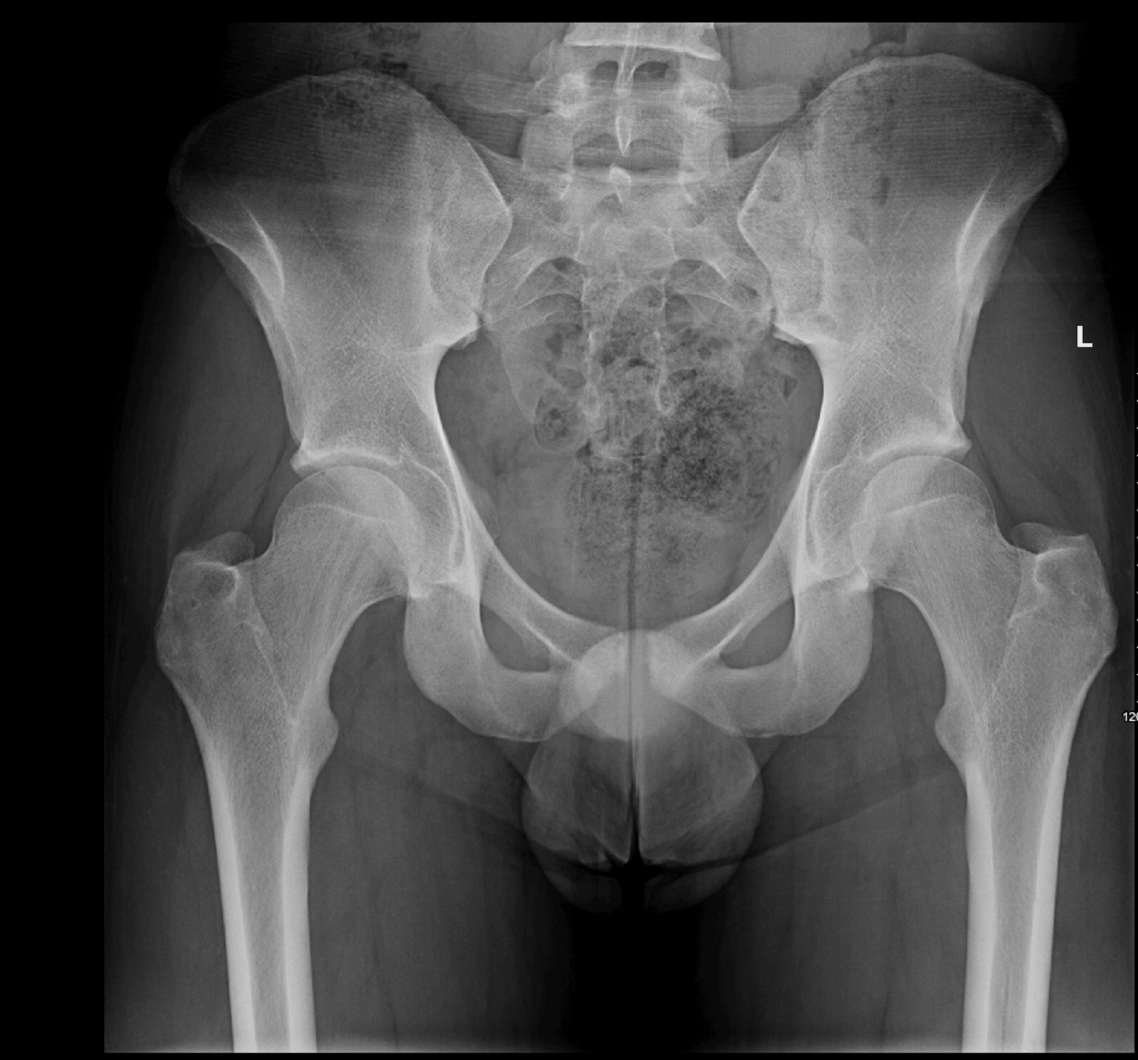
X-ray at the one-year follow-up Stable appearance of the osteitis pubis
